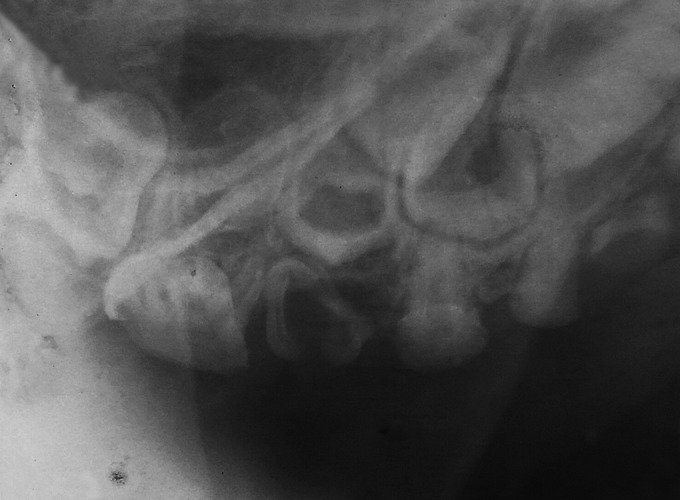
Intraoral periapical X-ray of upper right quadrant with marked cervical constriction and pulpal obliteration in deciduous teeth
